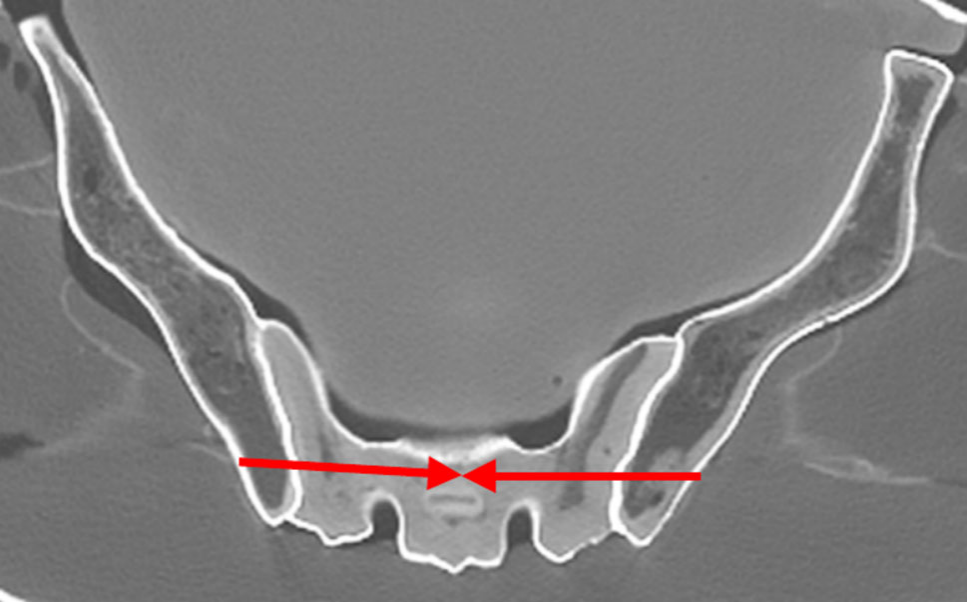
Axial CT image of the pelvis. Example for the S2 drilling corridor
